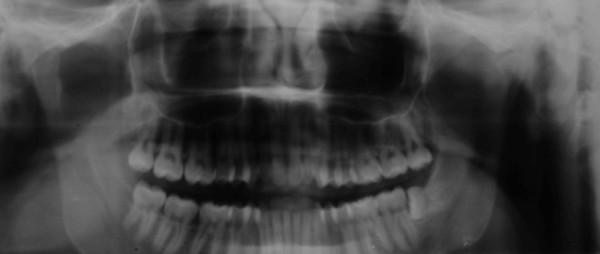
Panoramic radiograph revealing new growth in the left condyle after 18 months of therapy.
